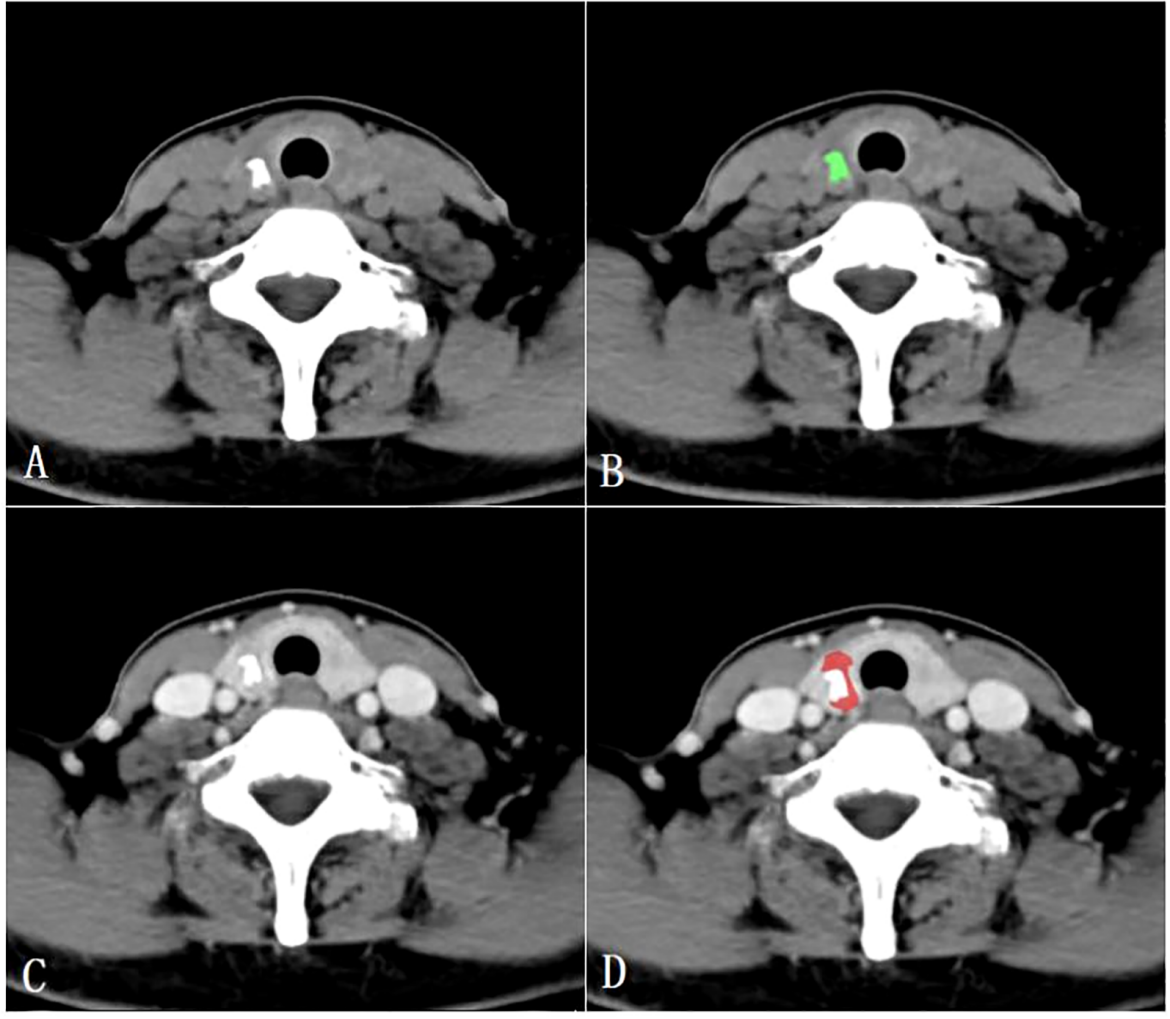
Region of interest placement in the calcified area of the papillary thyroid carcinoma on unenhanced CT images (A, B); Region of interest placement in the parenchymal area of the papillary thyroid carcinoma on venous phase CT images (C, D).
